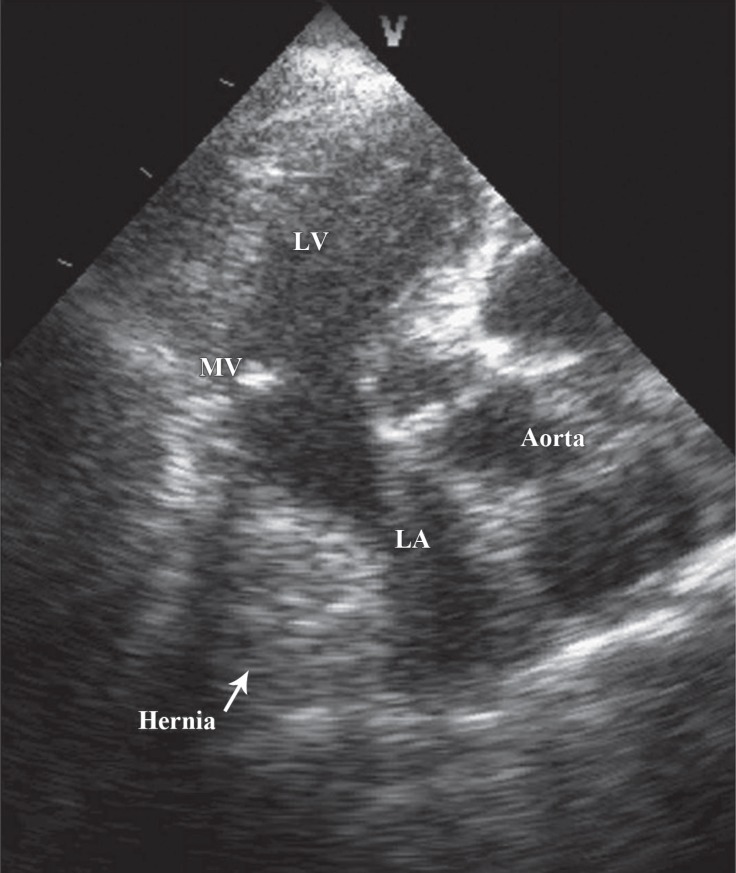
Hiatal hernias are described as abnormal protrusions of the stomach through the diaphragmatic esophageal hiatus. They are usually latent, with symptoms often related to gastroesophageal reﬂux signs. Huge hernias can rarely be misdiagnosed as intracardiac masses during echocardiography. Additionally, they can cause paroxysmal atrial fibrillation attacks due to symptomatic left atrial compression and the irritation of the vagus nerve. Huge hiatal hernias may mimic cardiac masses and rarely may cause paroxysmal atrial fibrillation attacks, as was the case in our patient. They should, be carefully differentiated from other cardiac pathologies.
